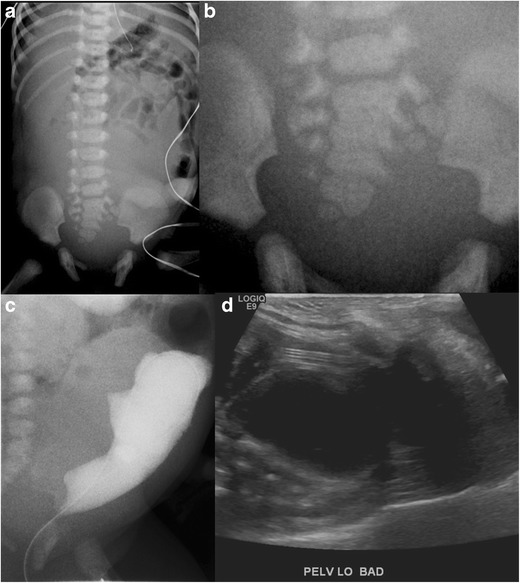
a-d: Dysmorphic sacrum with imperforate anus and presacral cystic mass: Frontal abdominal radiograph of a newborn demonstrates absence of bowel gas in the pelvis (*a*) with a history of no passage of meconium; the patient had ano-rectal atresia. Pelvic radiograph (*b*) demonstrates an abnormal sacrum. Retrograde cystogram (*c*) demonstrates an elongated urinary bladder with extrinsic indentations along its posterior aspect, suggesting a pelvic mass. Pelvic ultrasound (*d*) demonstrates a lobulated cystic pelvic mass; pathology was consistent with teratoma
